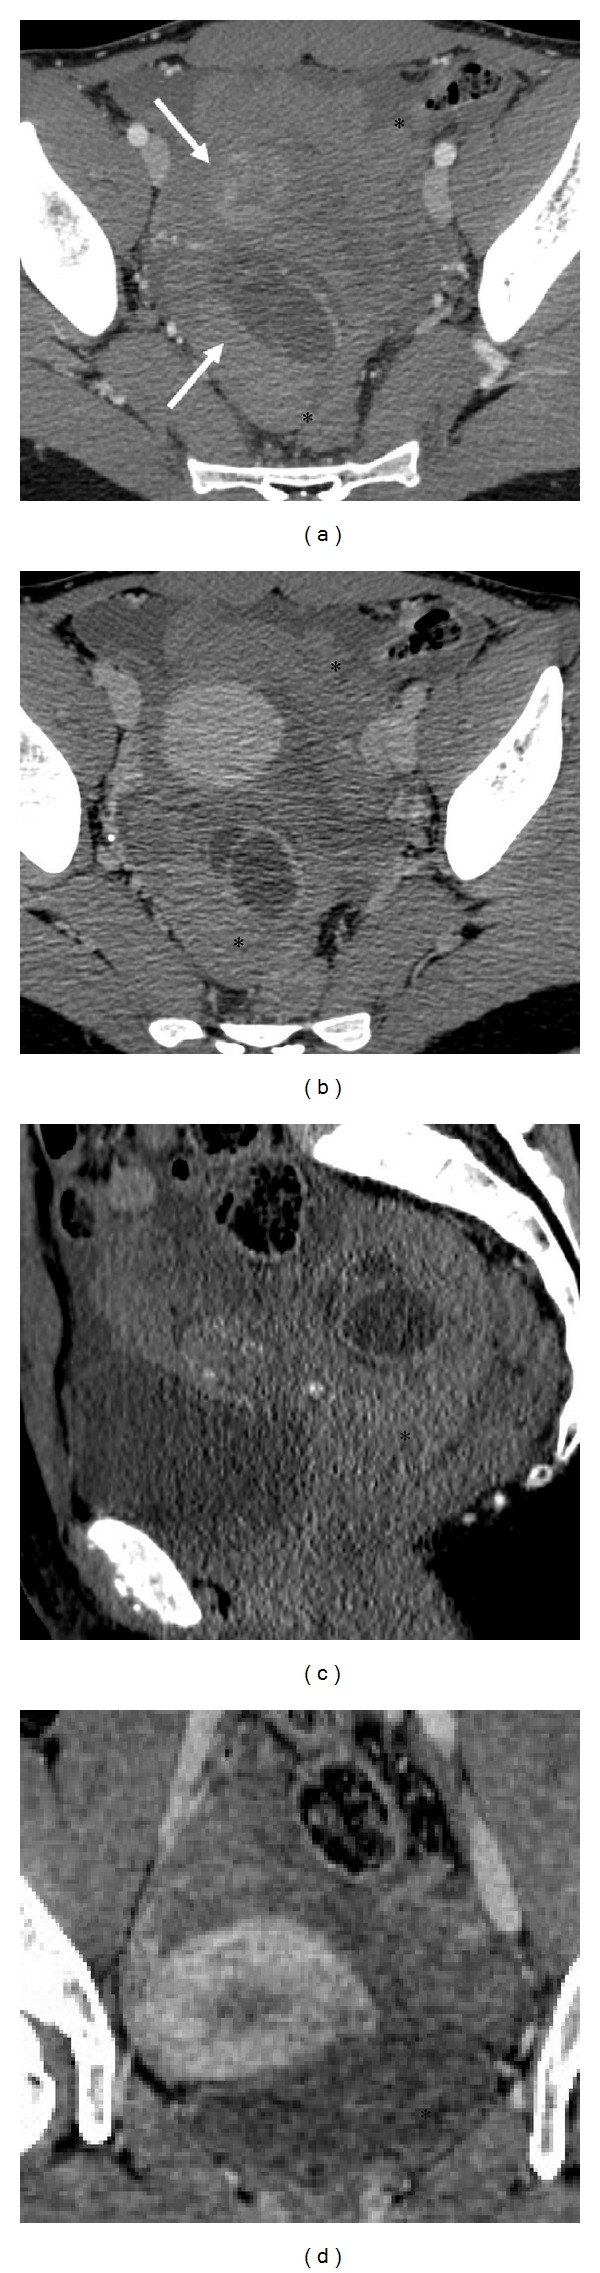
Dynamic contrast-enhancement CT scan. After injection of contrast medium pelvic CT images ((a, b) axial image; (c) sagittal image; (d) coronal image) show thickening and contrast-enhanced bilobate cystic wall in the right adnexa. The early arterial phase shows cloud-like extravasation from this lesion indicating active bleeding (arrows). Dynamic CT scan confirms diffuse hemoperitoneum (*).
